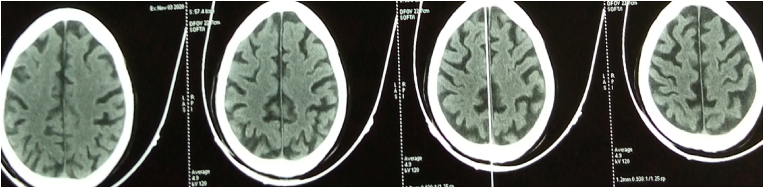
Brain CT scan showing diffuse cortico subcortical atrophy.
